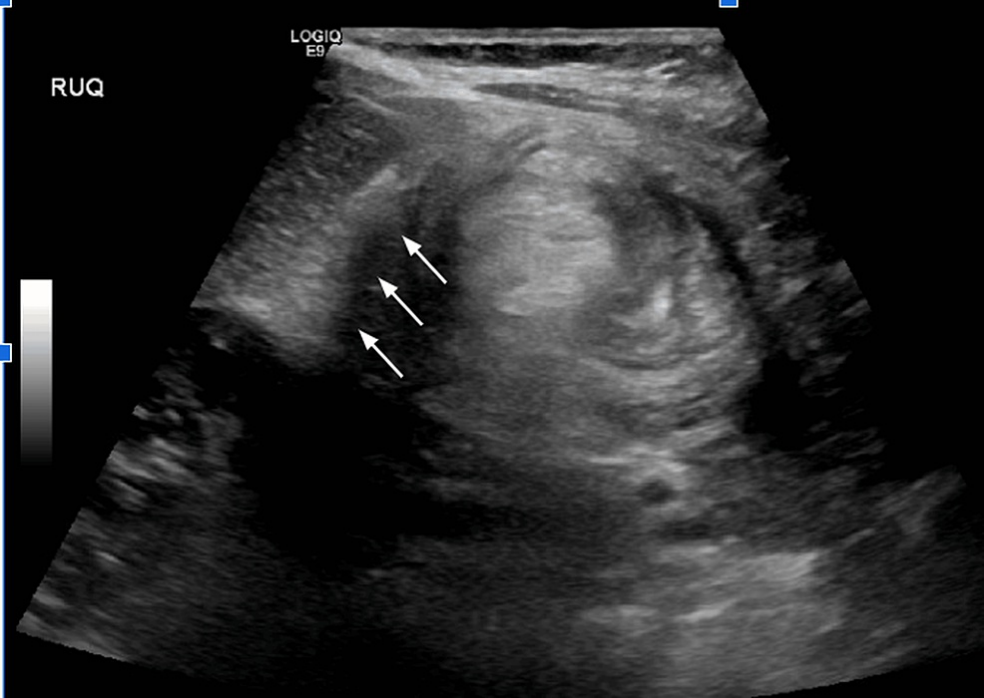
Abdominal ultrasound image four days after the first surgery The image shows recurrent ileocolic intussusception to the level of the hepatic flexure (white arrow).
